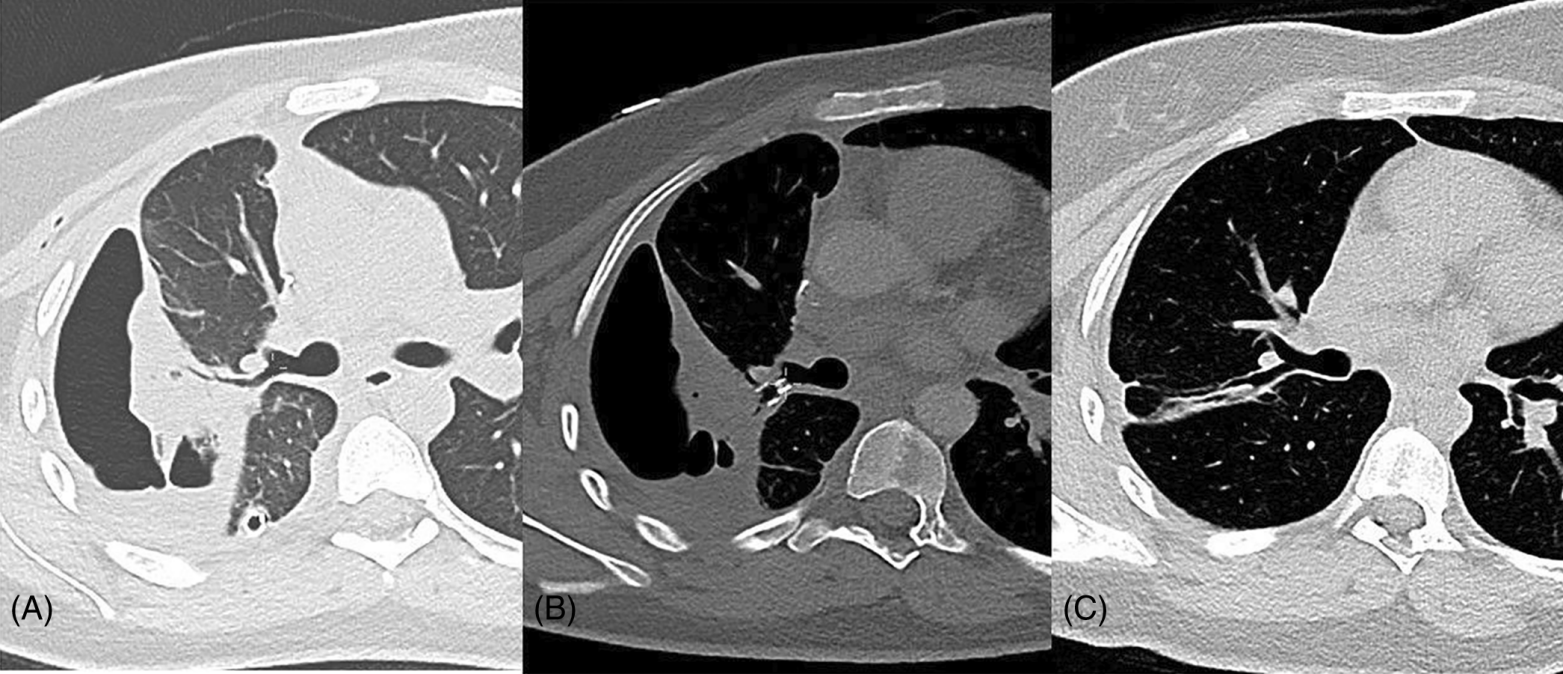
(A) Chest computed tomography (CT) scan showing necrotizing pneumoniae of the right upper lobe with a chest tube in place, before placing the endobronchial valve. (B) Chest CT scan showing a reduction of cavetating lesion of a necrotizing pneumonia of the right upper lobe 1 month after placement of endobronchial valve with the endobronchial valve in place. (C) Chest CT scan 1 year after placement en 6 months after the subsequent removal of the endobronchial valve, showing a limited area of atelectasis and minimal pleural thickening.
